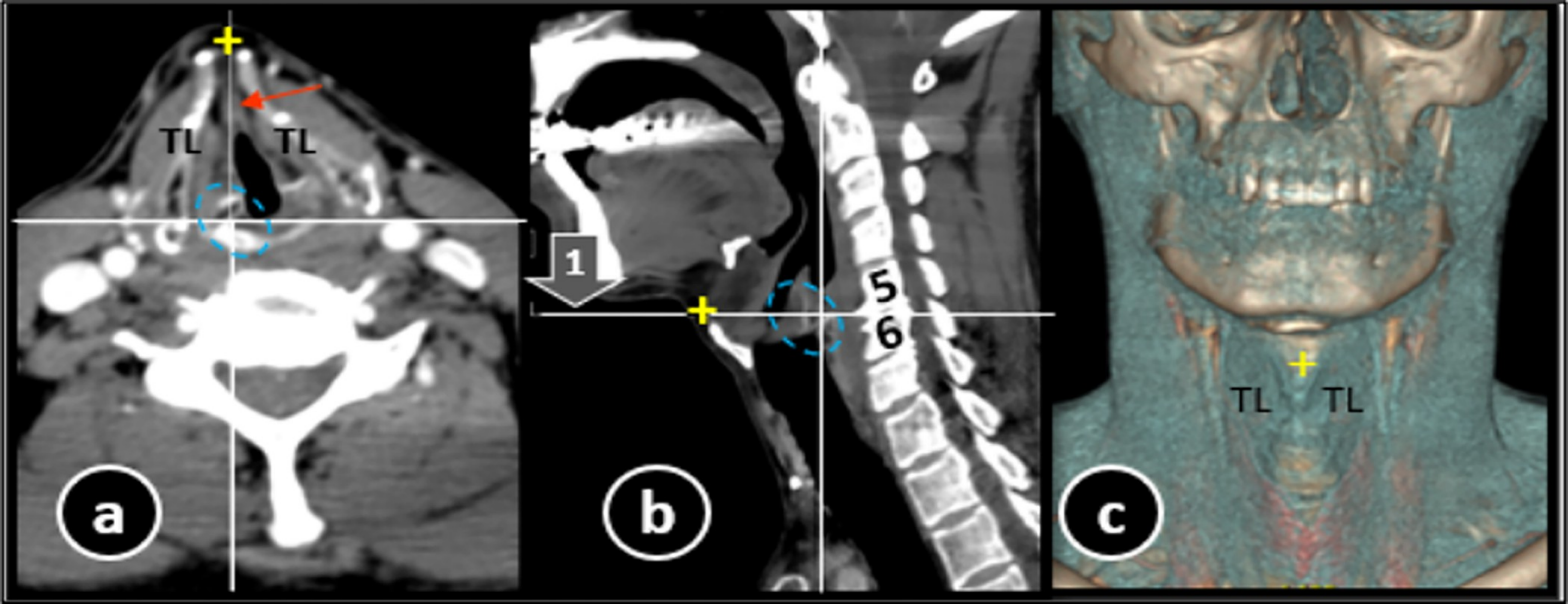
Axial (a) and sagittal (b) post-contrast CT neck showing glottis in non-parallel plane①. Split appearance of thyroid laminae (TL) shown by cross linking (+) this gap in a, b and 3D CT volumetric neck reconstruction. It is in actual a thyroid notch (c). Adipose tissue (red arrow), cricoarytenoid joint (dotted oval) and oblique crossing of plane① through vertebrae (5, 6) are also shown.
